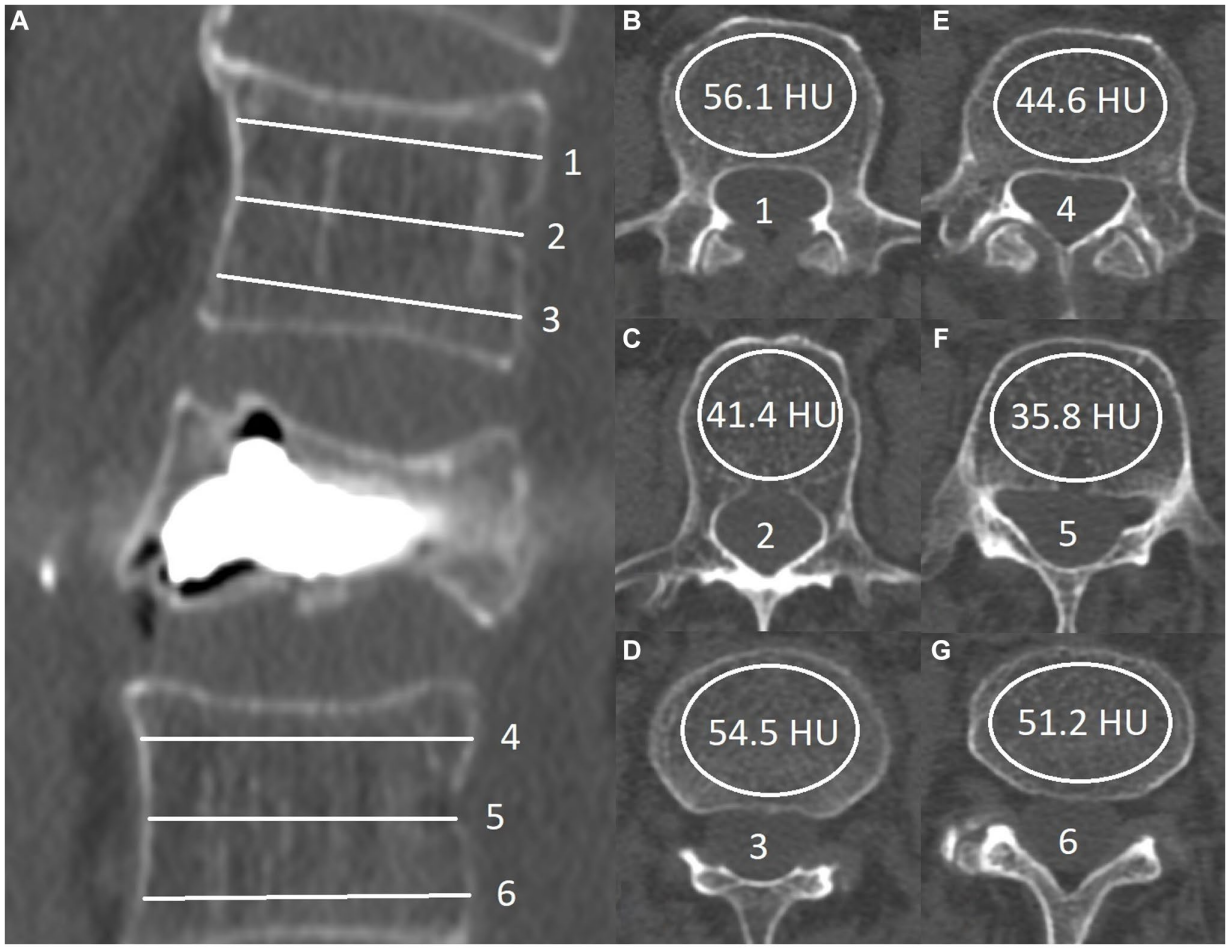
Sagittal view of postprocedural computed tomography: (A) Six transverse lines represent the level of the ROI measurement (B-G). HU_mean_ values are calculated by the formula (56.1 + 41.4 + 54.5 + 44.6 + 35.8 + 51.2)/6 = 47.3. The HU_min_ value in this patient was 35.8 (F).
